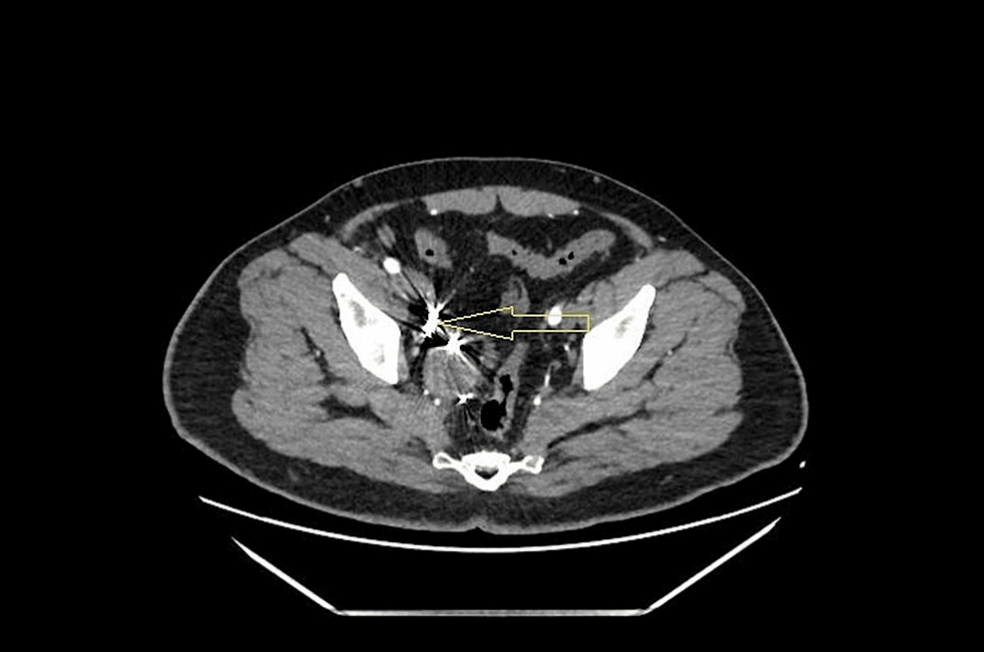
CT angio at the three-month follow-up The yellow arrow shows an aneurysm smaller in size (5.2 x 4.7 mm) with blood flow inside not enhancing with multiple small artifacts at its small narrow neck (indicating successful embolization) CT: computed tomography
